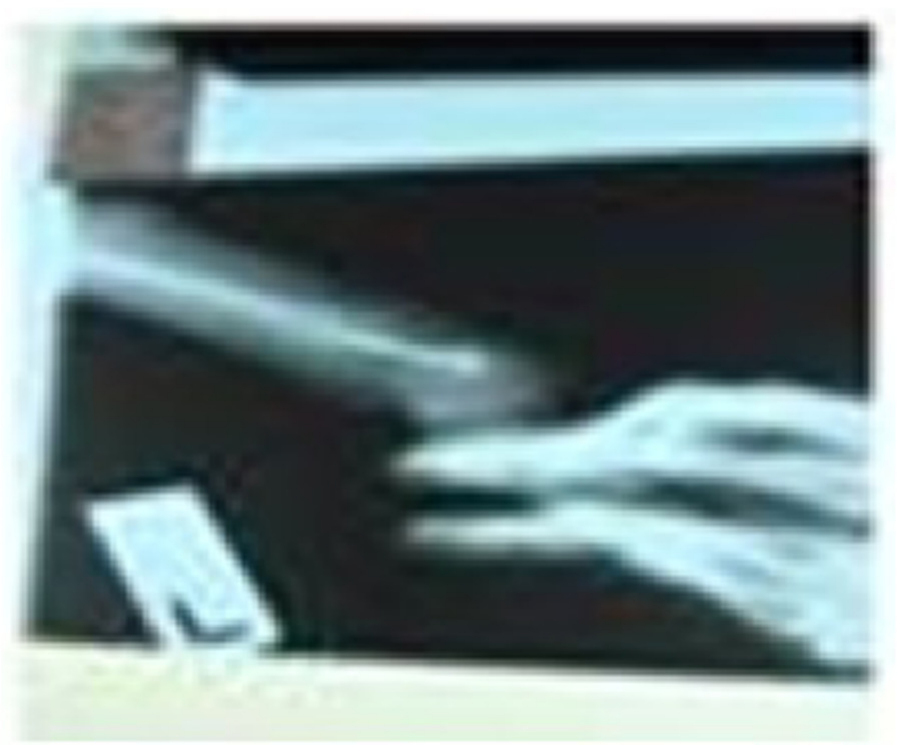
Plain X-ray of the left upper limb
